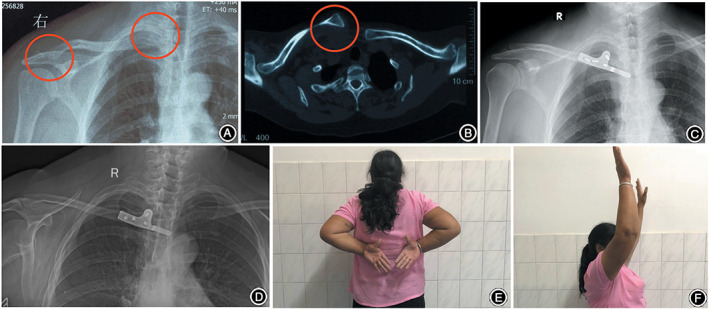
In patient 4 (54‐year‐old woman), the (A) preoperative radiograph and (B) CT scan showed a right anterior dislocation of the sternoclavicular joint (SCJ) and an ipsilateral Rockwood type IV acromioclavicular joint dislocation. (C) A postoperative radiograph after Balser plating showed a good reduction of both the SCJ and acromioclavicular joint. (D) During a follow up of 13 months, the patient showed no recurrent dislocations of the sternoclavicular joint or acromioclavicular joint and developed no hook migration. (E, F) Although the patient had mild shoulder function limitation, she was satisfied with the treatment.
